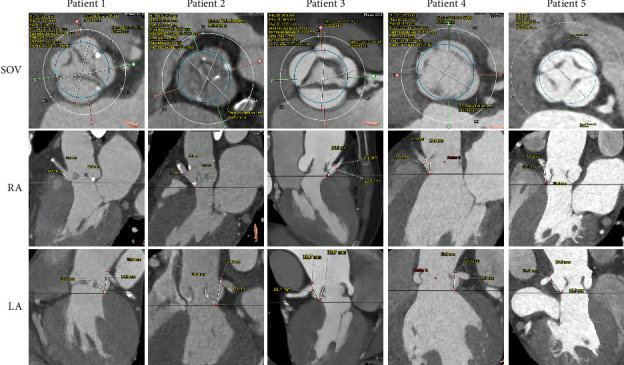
The dimensions of the aortic sinus, coronary artery, and valve leaflet of the QAVs of five patients were measured by CTA. (SOV) Measurements of the anticipated morphology of the implanted valve after release at the aortic sinus level and the diameters of right coronary sinus (RC) and left coronary sinus (LC); (RA) Assessment of right coronary artery (RCA) occlusion risk, including the heights of the RCA and the lengths of the adjacent valve leaflet; (LA) Assessment of left coronary artery (LCA) occlusion risk, including the heights of the LCA and the lengths of the adjacent valve leaflet.
